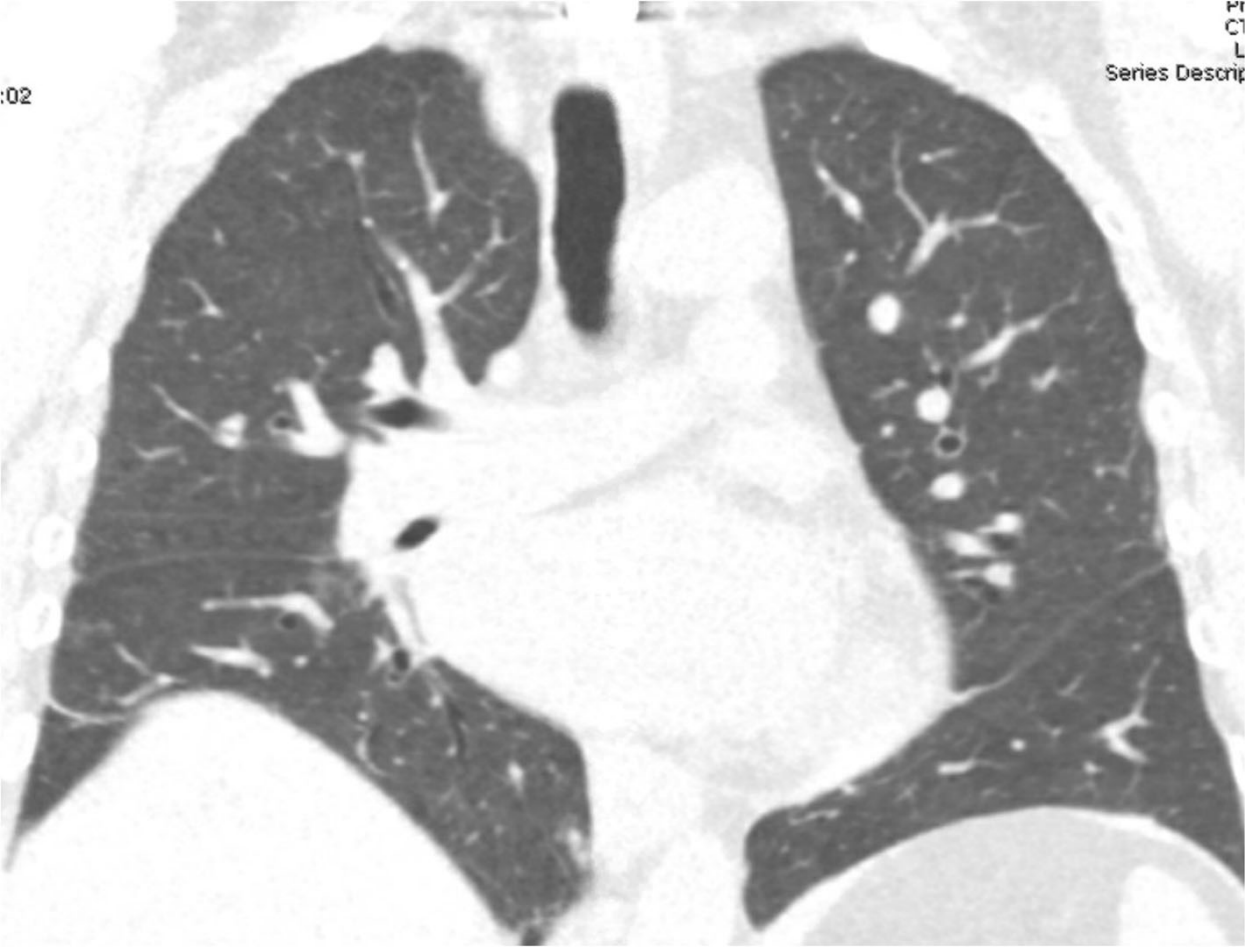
Coronal CT reformatted image of the chest during the admission for the recent viral infection (before Covid) shows clear lungs without any cysts and no evidence of infiltrate
